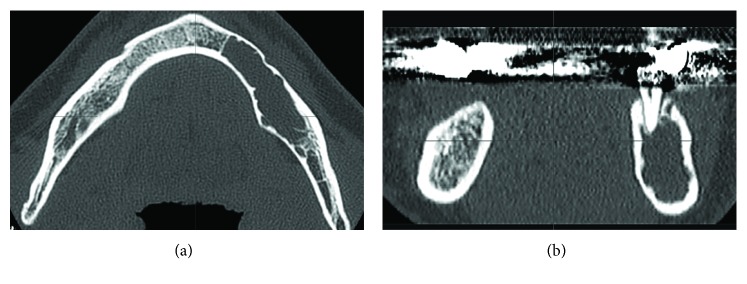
Computed tomography (CT) image before surgery. The axial (a) and coronal (b) CT images show tumor infiltration of cortical bone extending to the inferior mandibular border.
